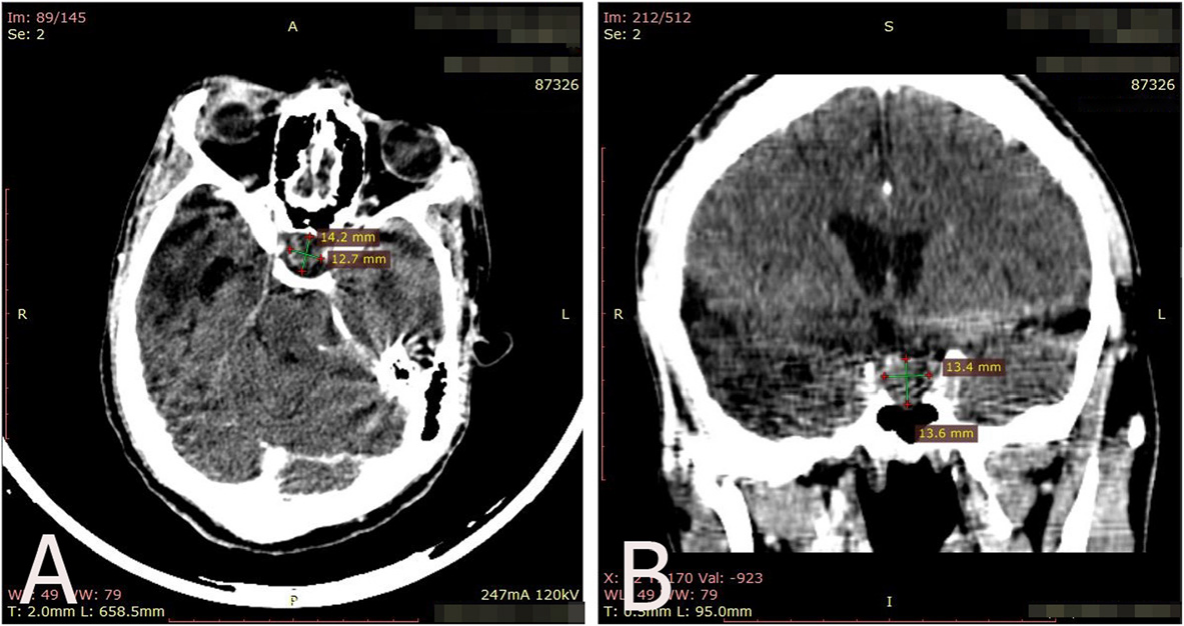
Control computed tomography scan taken 8 months after head trauma. Axial (A) and coronal (B) computed tomography scans show a reduction of the pituitary size with no evidence of a tumor.
